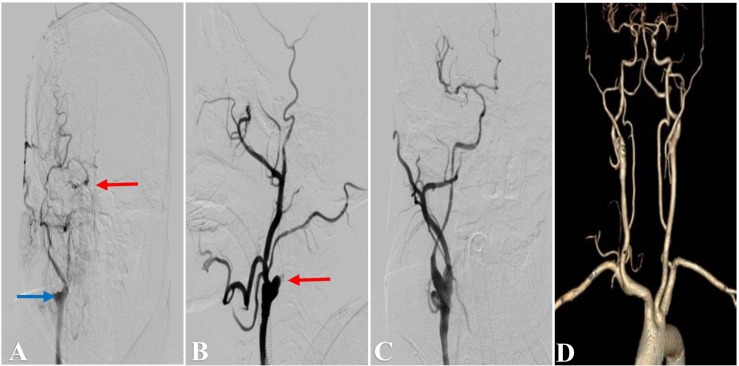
Representative Case 2. A 51-year-old man with left-sided limb weakness underwent hybrid surgery for internal carotid artery occlusion (ICAO). (A) Preoperative angiography showed right ICA occlusion (blue arrow) with filling at the cavernous segment (red arrow). Intraoperative angiography showed (B) an artificial stump after carotid endarterectomy (red arrow) and (C) successful recanalization after deployment of the stent. (D) Computed tomography angiography showed a patent ICA at 3 months after the surgery.
